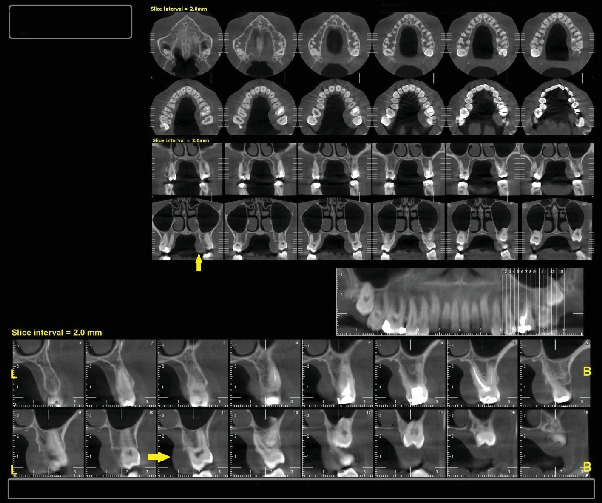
CBCT of the patient's maxilla in axial, coronal, and cross-sectional cuts. Yellow arrows show the soft tissue bulge in the palate with no invasion to sinus or nasal cavity; a mild corrosion of the lingual cortex of the maxillary alveolar bone is evident.
